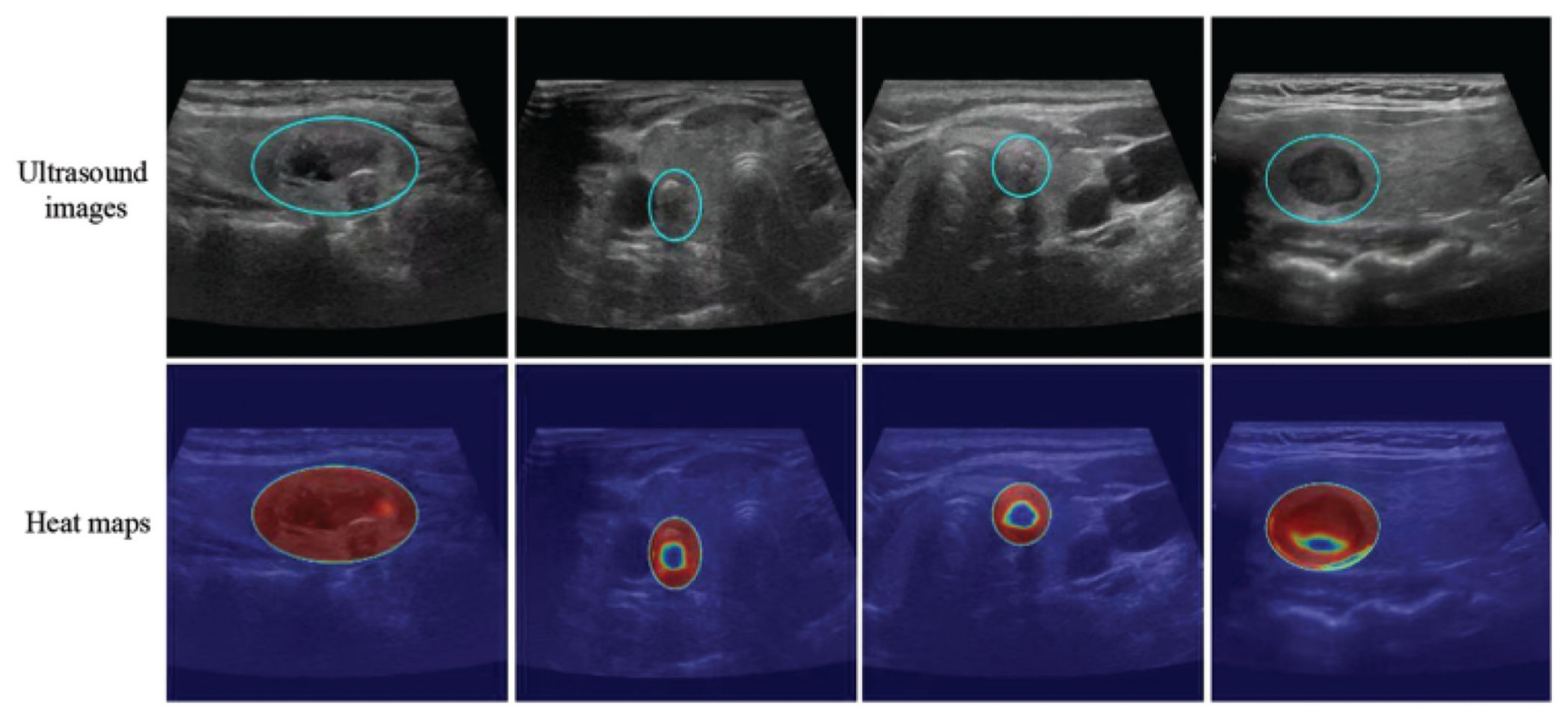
Localization of thyroid nodules. The blue circle is the location of the thyroid nodules, and the heat map represents the visualization results. Each column shows the same ultrasound image. The cold tone region of the visualization image is the most important part that our model could recognize
